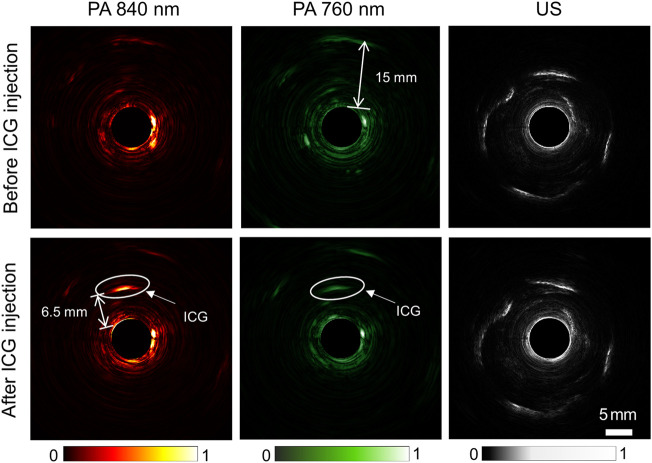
Comparison of photoacoustic and ultrasound images of the rabbit rectum before (top row) and after (bottom row) ICG injection. From left to right, the three columns depict photoacoustic imaging results with 840 nm and 760 nm wavelengths and ultrasound imaging results, respectively.
